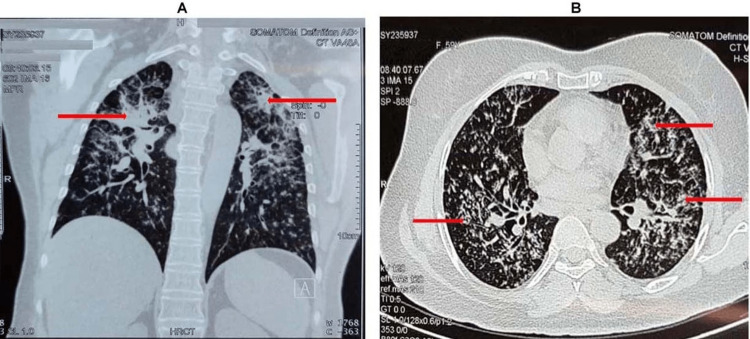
High-resolution CT scan of the chest showing diffuse reticular opacity affecting all segments of both lungs A, coronal view; B, axial view
